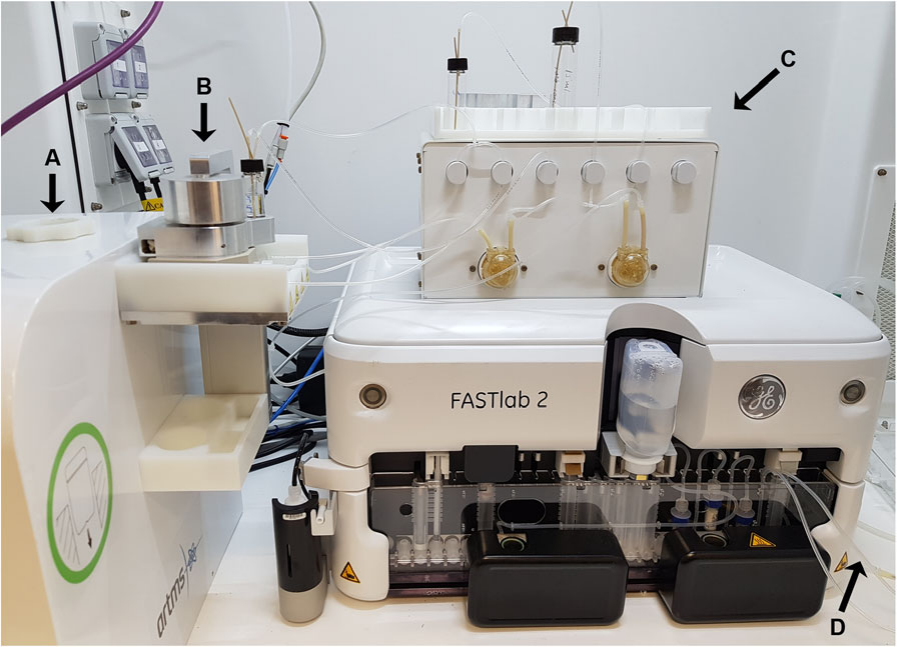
ARTMS receive station (a), zinc-68 dissolution cell (b) and dissolution box (c) coupled to the GE FASTlab 2 Developer synthesis module with gallium-68/zinc-68 separation cassette installed. The [^68^Ga]GaCl_3_ outlet (d) was connected to the Modular-Lab PharmTracer (not shown) for automated radiolabelling of PSMA-11
